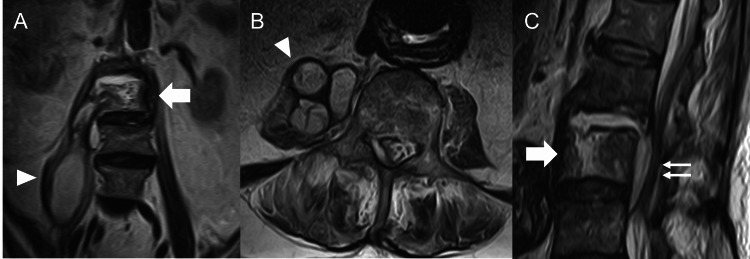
Preoperative magnetic resonance imaging of lumbar spine (A) coronal image, (B) axial image, (C) sagittal image T2-weighted magnetic resonance imaging showing multifocal right iliopsoas (arrowheads), epidural abscesses (double arrow), and erosion of the L3 vertebral body (arrows)
